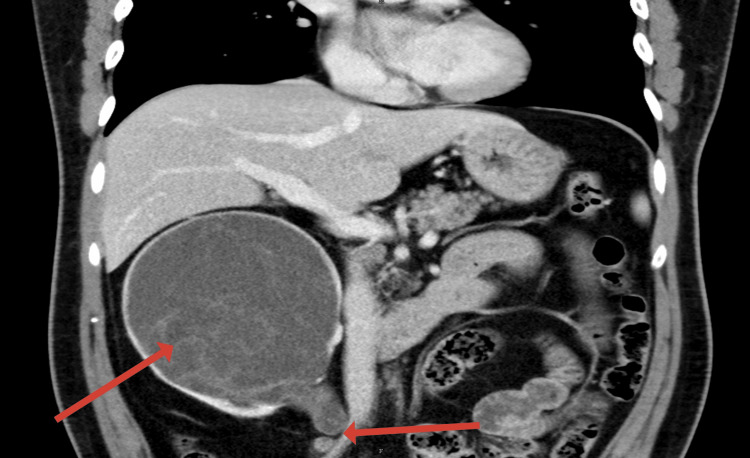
Coronal CT image demonstrating the abrupt tapering of the right proximal ureter at the iliac crossing, suggestive of distal ureteric stricture. The first arrow points to the enlarged right kidney, which has a cystic appearance, and the second arrow points to tapering of the ureteric segment, which was suspected to represent a stricture.
